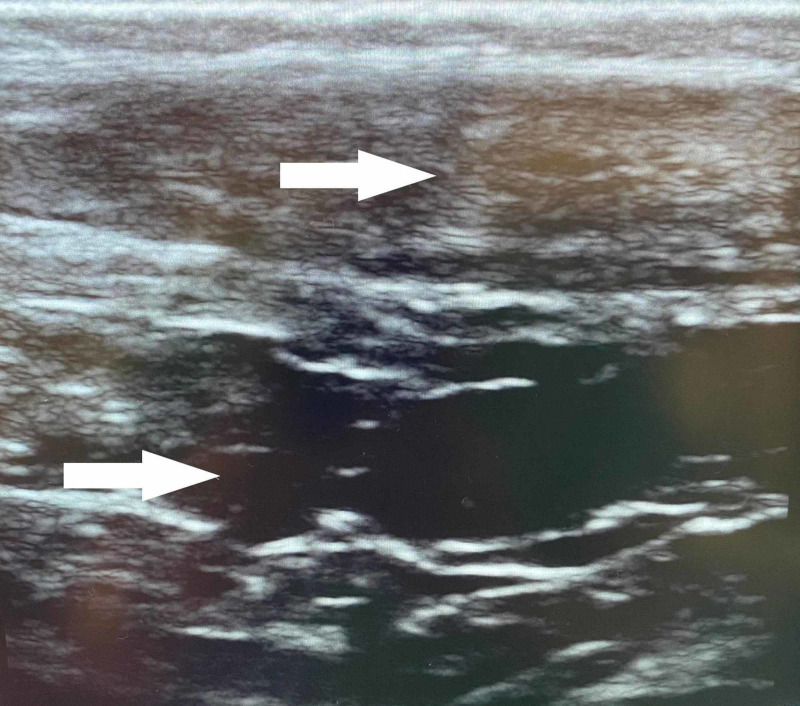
Longitudinal Ultrasound Achilles Tendon Tear Longitudinal sonographic imaging of Achilles tendon with midpoint defect (upper white arrow) with anechoic fluid posteriorly (lower white arrow) suggestive of hemorrhage and partial versus full tendinous tear.
